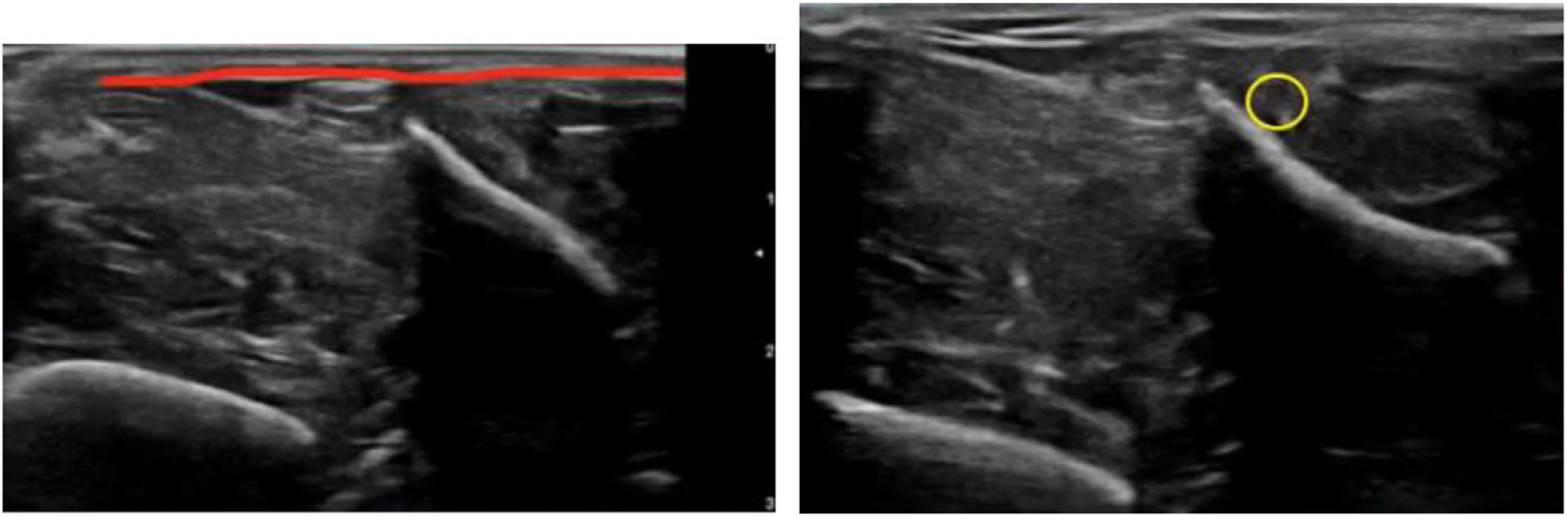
Ultrasound view. The fascia is indicated in red. The yellow circle indicates the superficial fibular nerve.
